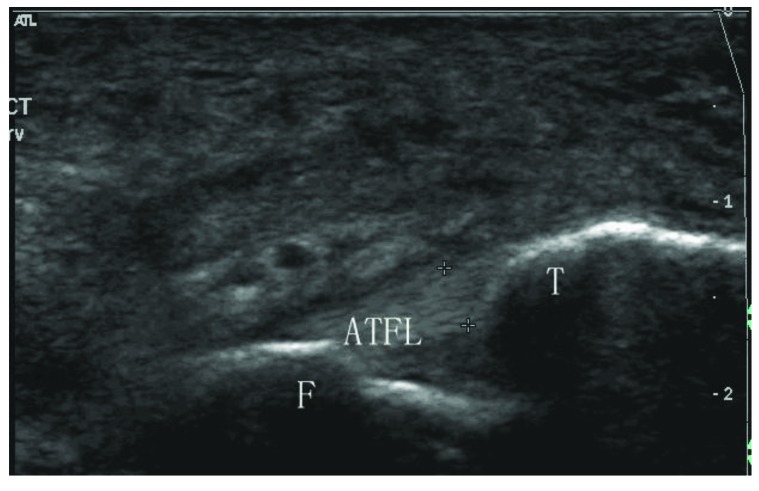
Sonogram shows the ligament thicker than 2.4 mm wide (type IV). ATFL: anterior talofibular ligament; F: fibula; T: talus.
